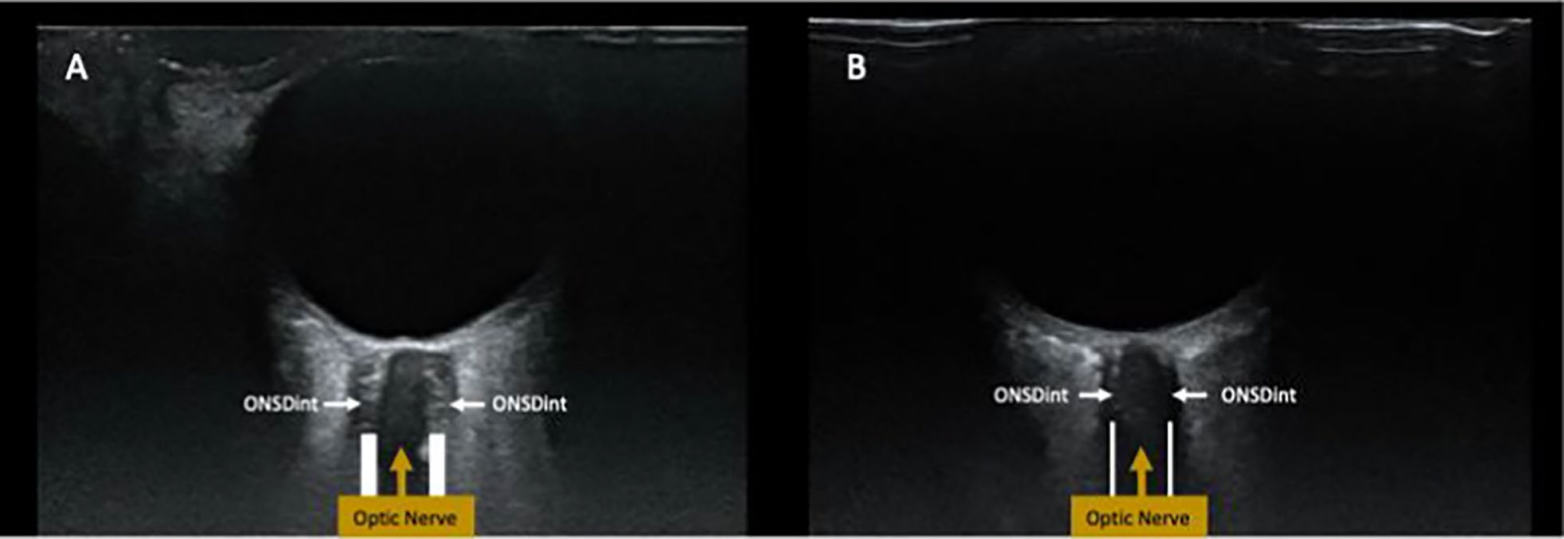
Optic nerve sheath ultrasound. Two examples of optic nerve sheath images: A in a patient with intracranial haemorrhage secondary to an arterio-venous malformation, B in a healthy individual. The ONSDint (as per Hirzallah et al [66]), is denoted by the outer border of the radiolucent parallel lines shown and annotated. ONSDexternal corresponds to the wider, outer borders, of the outer dark parallel lines (not annotated). 3 mm depth is not shown, but is important for performing correct diameter measurement
